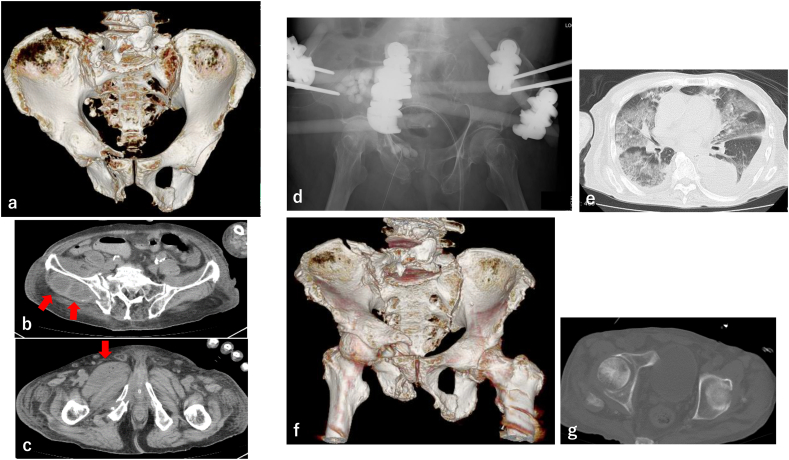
Radiographs and computed tomography (CT) of the pelvis and lung (a) Three-dimensional (3D) CT shows right iliac fracture, sacroiliac joint dislocation, and right pubic fracture classified to AO/OTA classification 61C1.2 and (b, c) abscess formation in the right hip and adductor muscle (red arrow). (d) Plain radiograph after continuous local antibiotics perfusion (CLAP), antibiotic-impregnated cement implantation, and external fixation of the pelvic fracture. (e) Bilateral infiltrates, indicative of eosinophilic pneumonia. (f) 3DCT of the pelvis 2 months after injury show deformation and nonunion and (g) no emphysematous cystitis. (For interpretation of the references to colour in this figure legend, the reader is referred to the web version of this article.)
